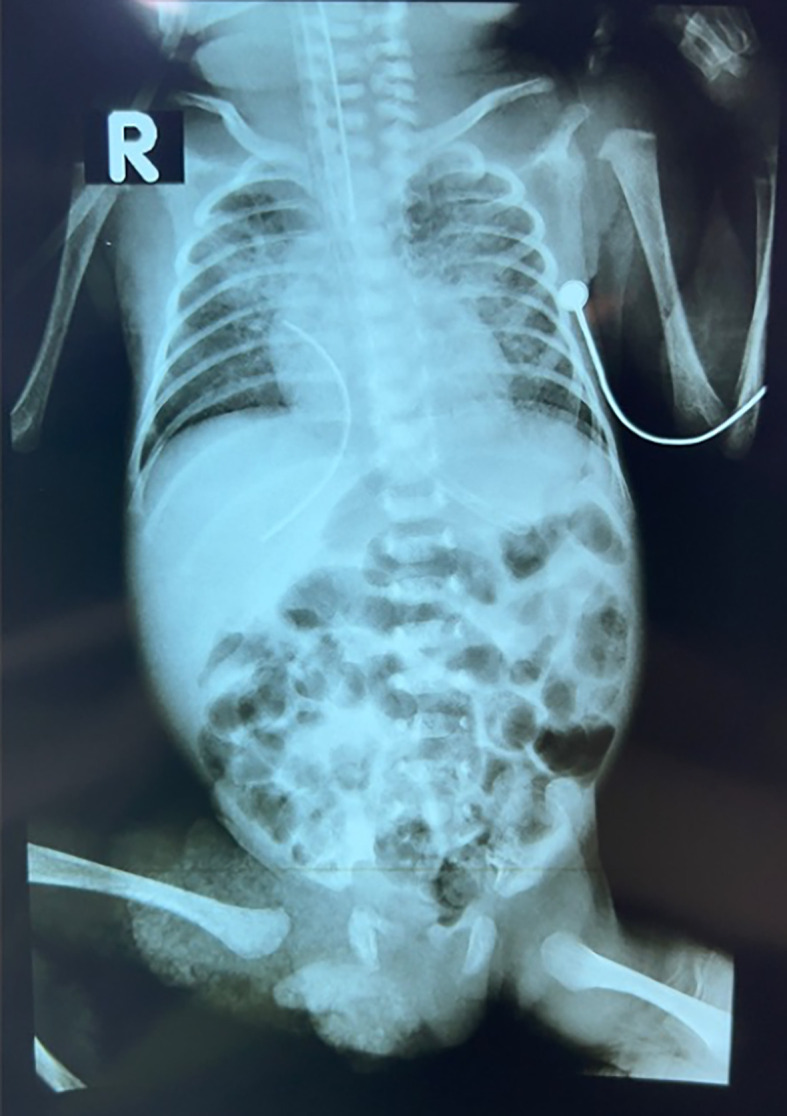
The preliminary thoracoabdominal X-ray image showing a radiopaque catheter at the right paravertebral region.
